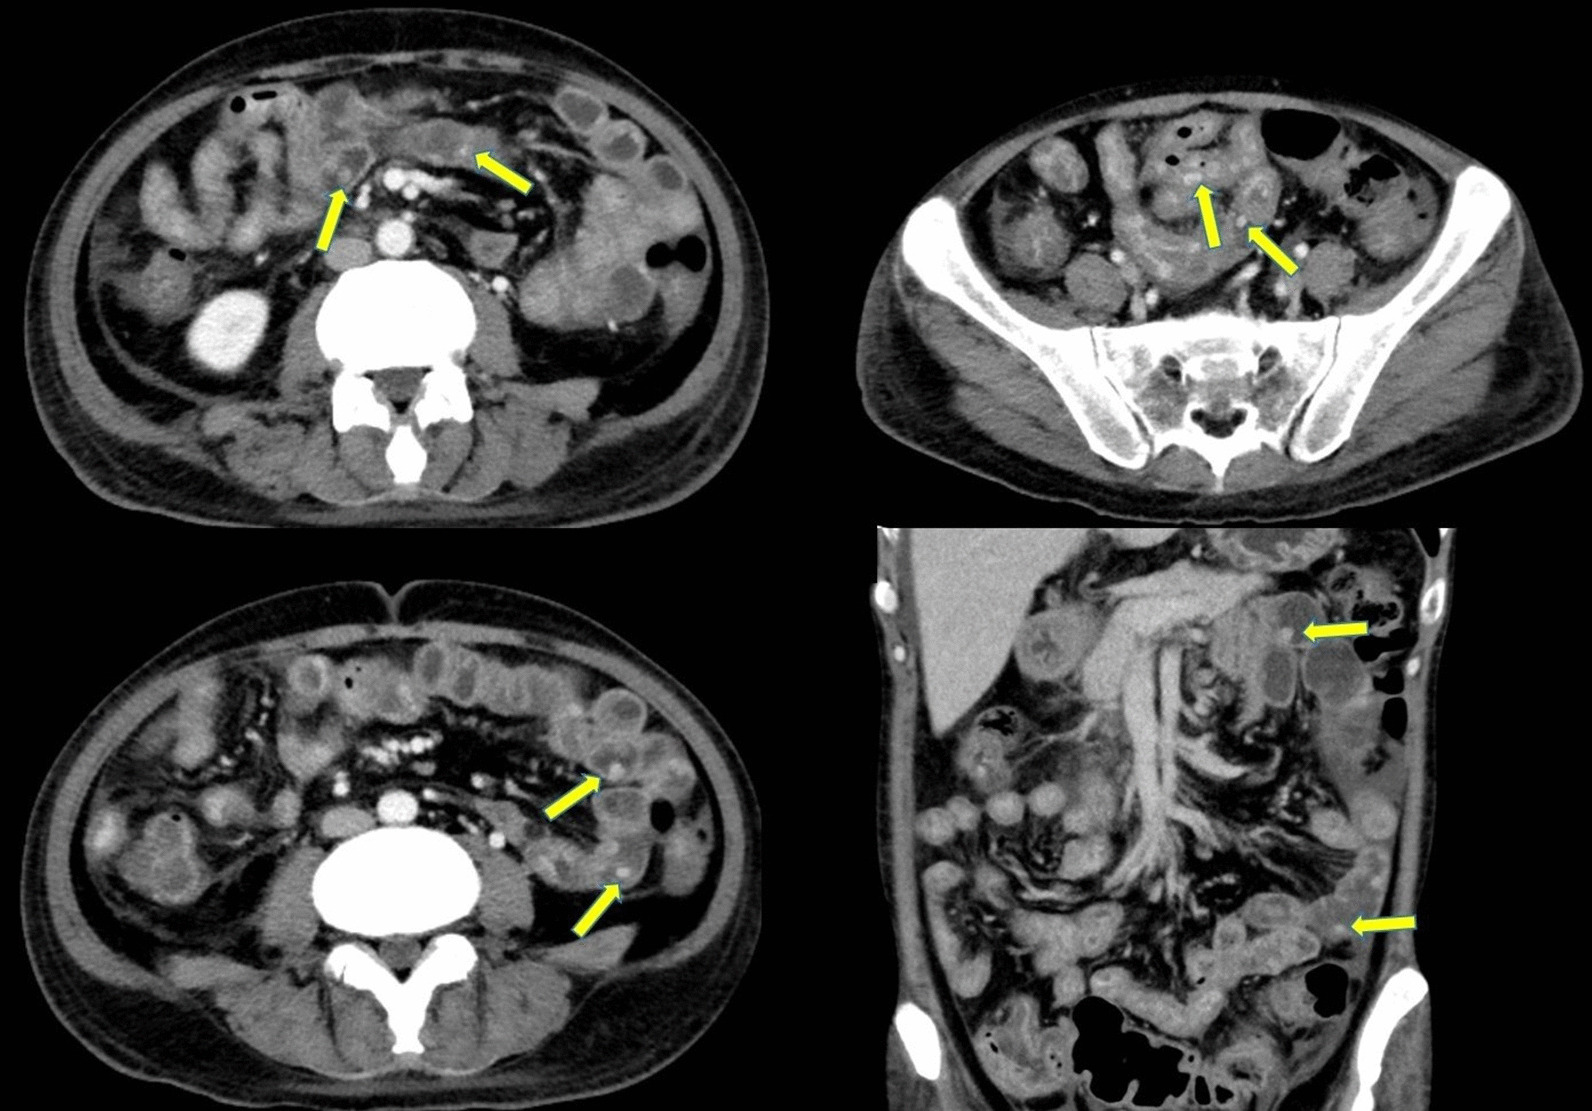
Abdominal CT scan revealed diffuse edematous change of gastrointestinal tract and multiple hyperdense nodular lesions in the small bowel (arrows)
